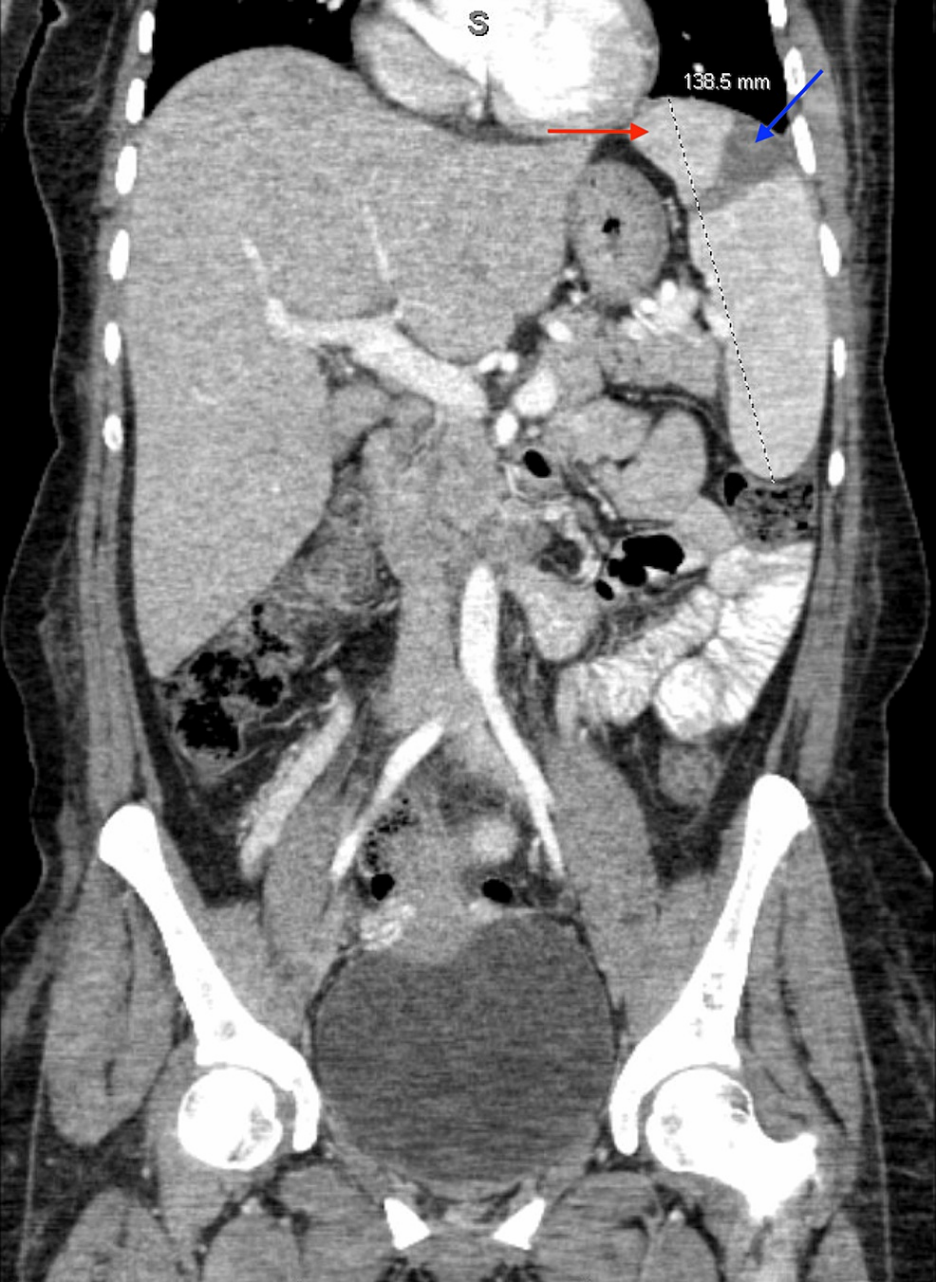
CT scan of the abdomen and pelvis CT scan of the abdomen and pelvis in coronal view demonstrates an enlarged spleen measuring 13.8 cm (red arrow) with a band of hypoattenuation that likely represents an infarction (blue arrow).
